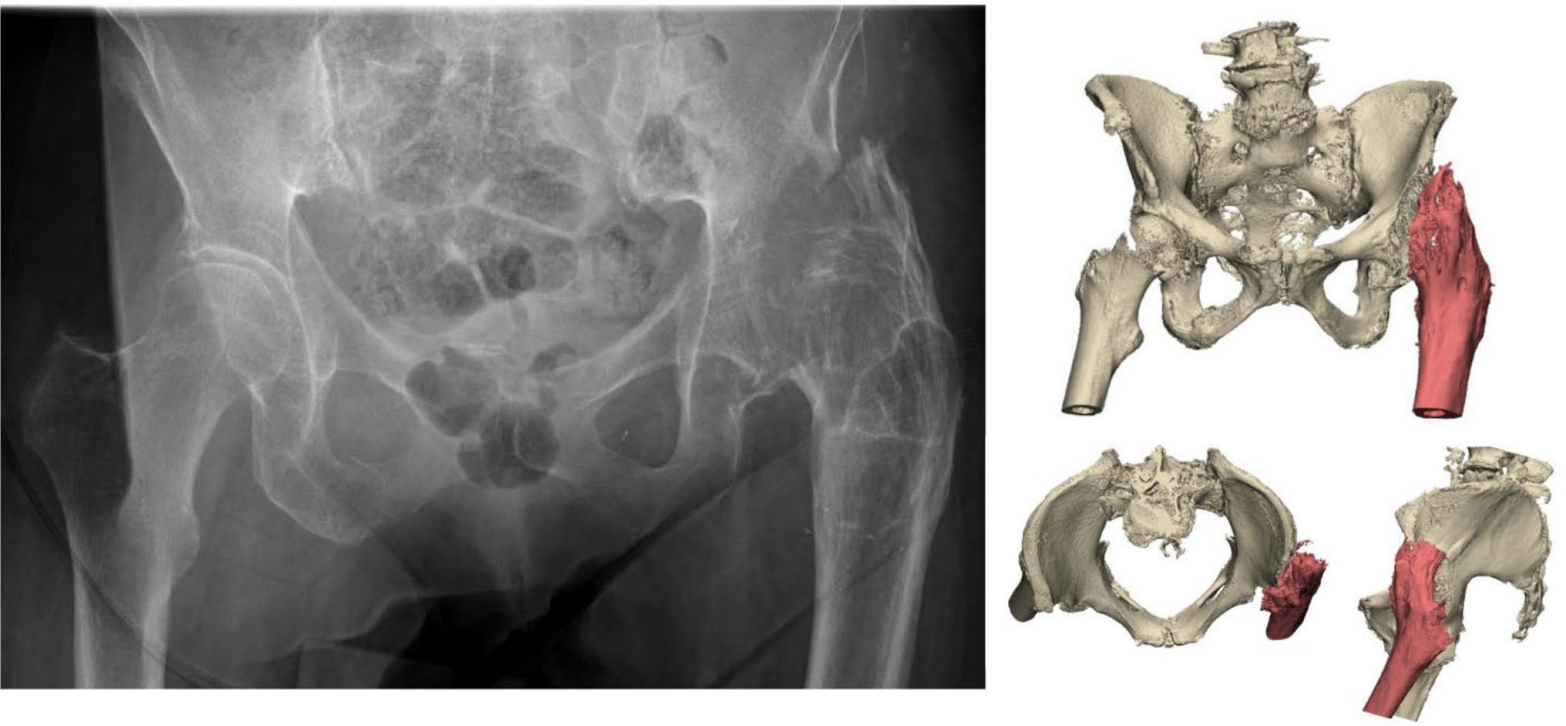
Case 1. Preoperative X-ray and 3D reconstruction, a fracture at the level of the pelvis/acetabulum of the left arthrodesed hip
